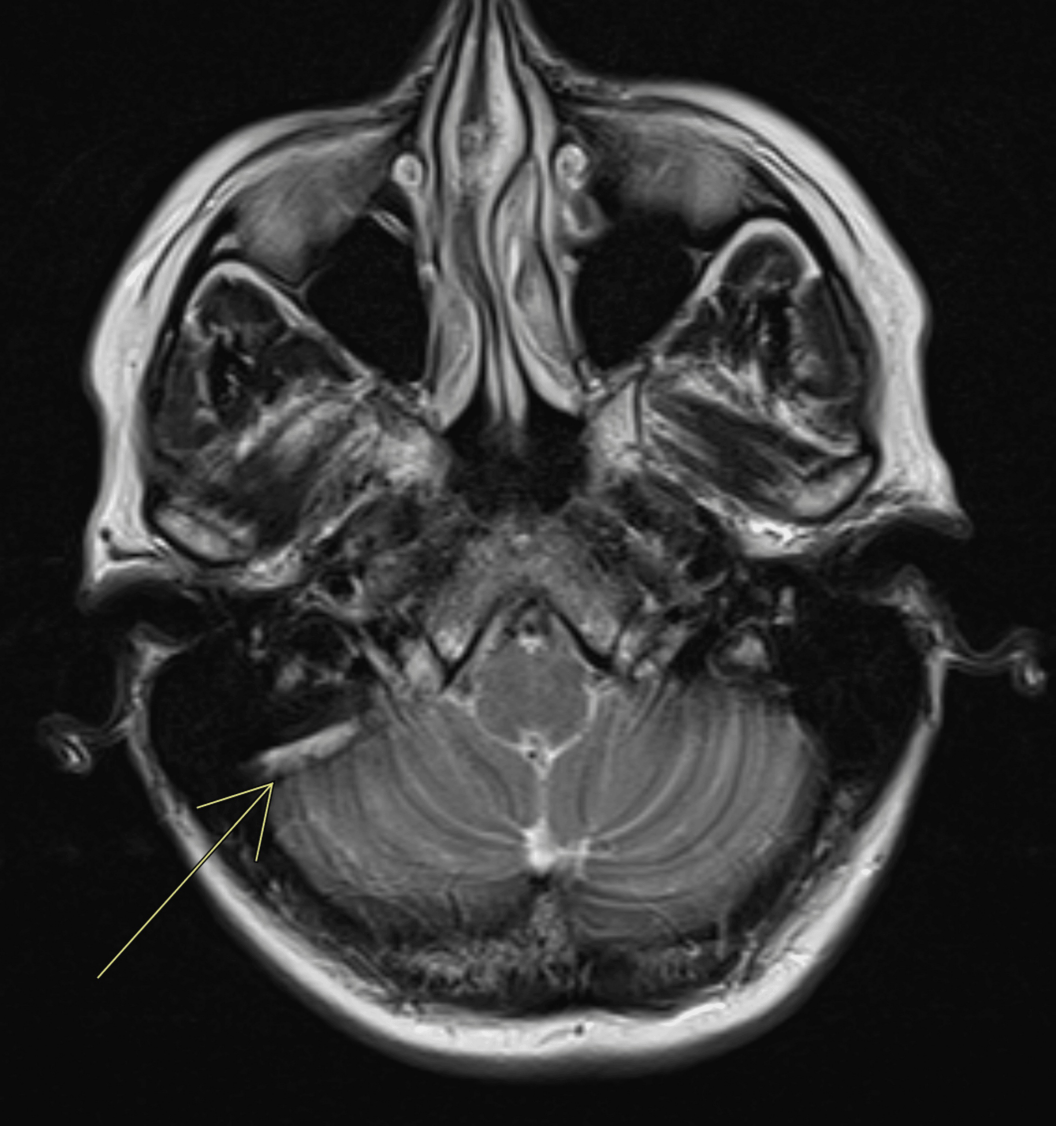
MRI head with contrast (axial T2) at the level of sigmoid sinus showing a chronic thrombus (linear hyperintensity). The white arrow indicates the chronic thrombus.
